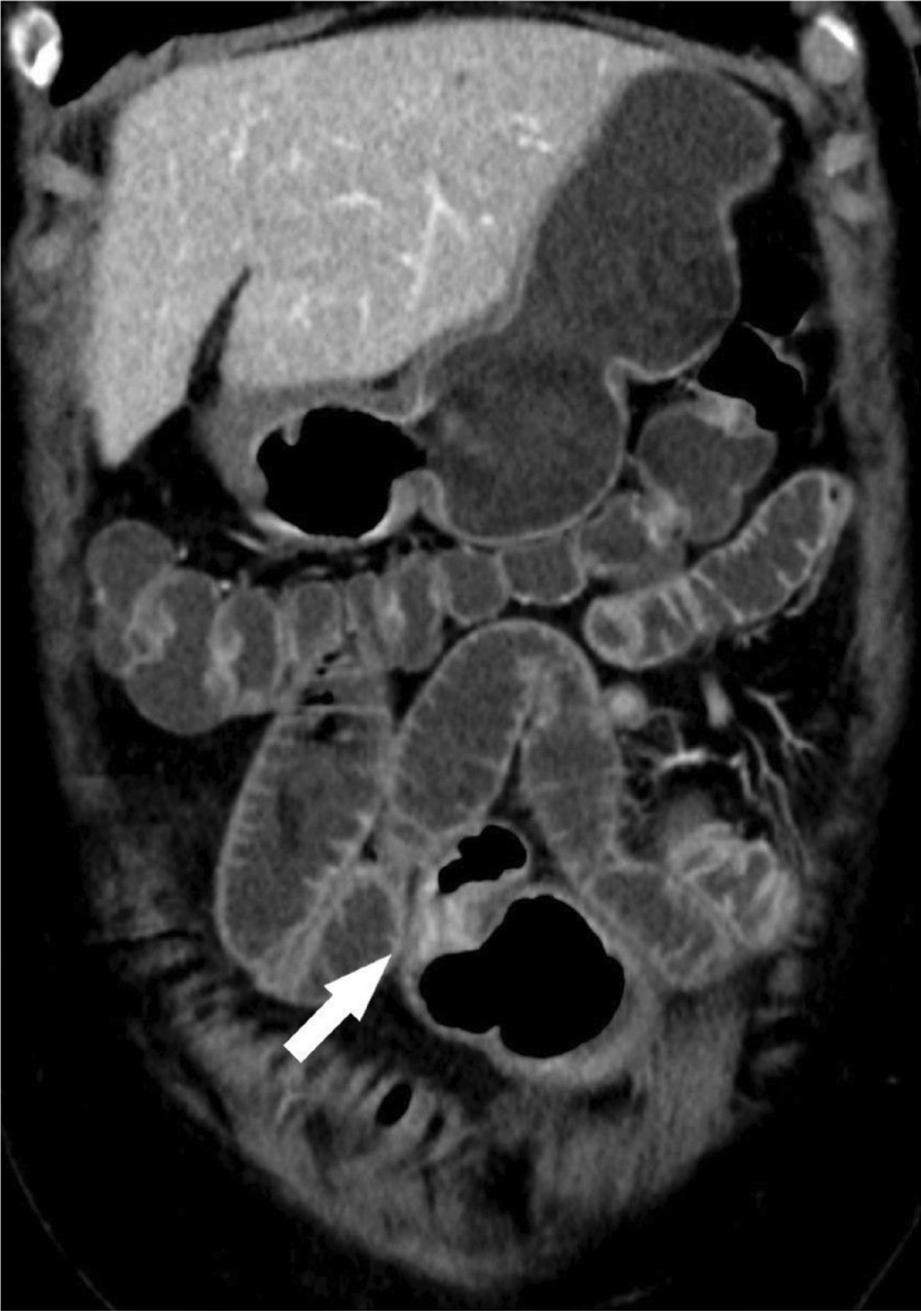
CT showing stenotic ileal loop with marked contrast-enhancing wall thickening.
